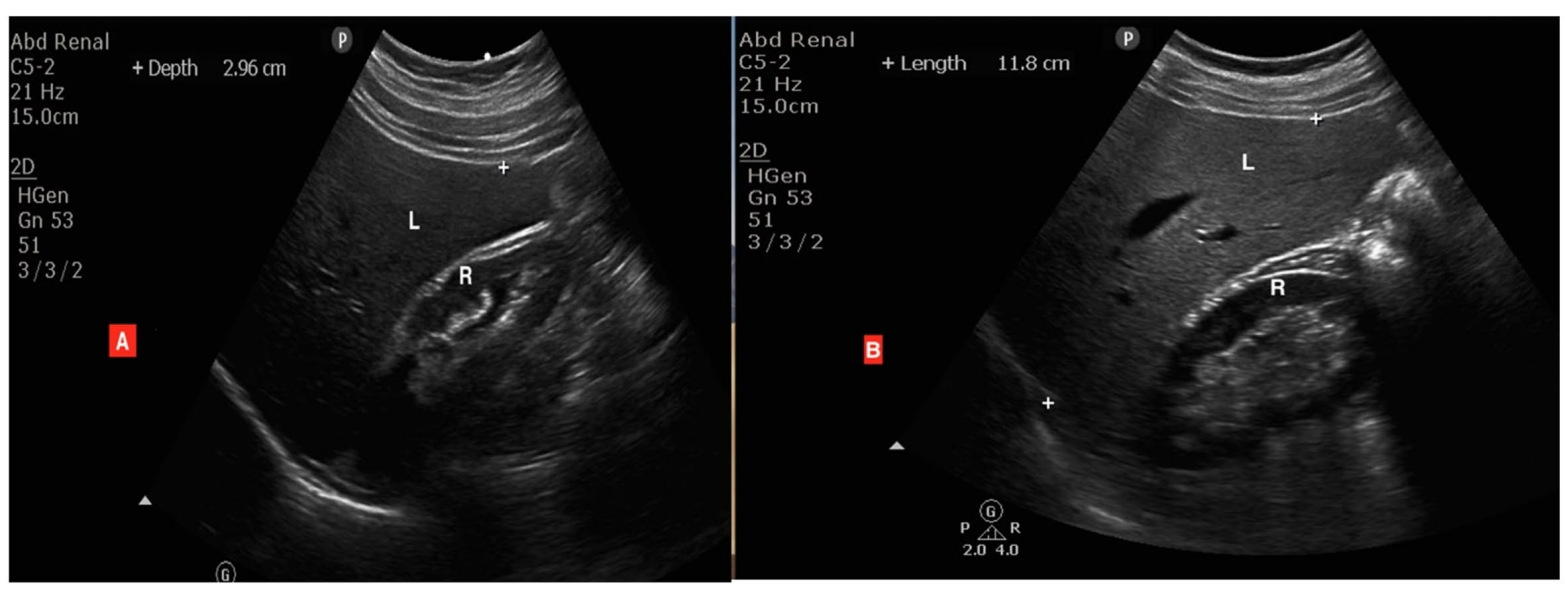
Ultrasound images of two different cases: (A) a normal liver with similar brightness of the liver (L) and renal cortex (R), while (B) is a patient with grade 1 (mild) fatty changes showing the liver (L) is brighter than the renal cortex (R) but the wall of the intrahepatic vessels is still clearly seen.
